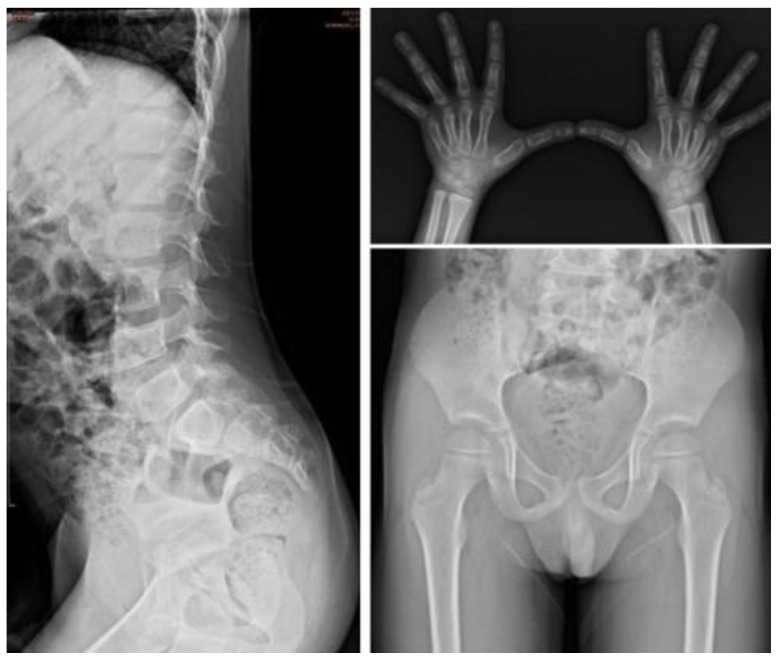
The bone X-rays at 6.1 years of age of subject No. II-8 who had the variation alleles c.103 + 34_56dup; c.684A > G [p.Pro228=]; c.851C > T [p.P284L]; c.1180 + 184T > C. At 6.1 years of age, X-rays of his spine, hands, and pelvis were all normal.
